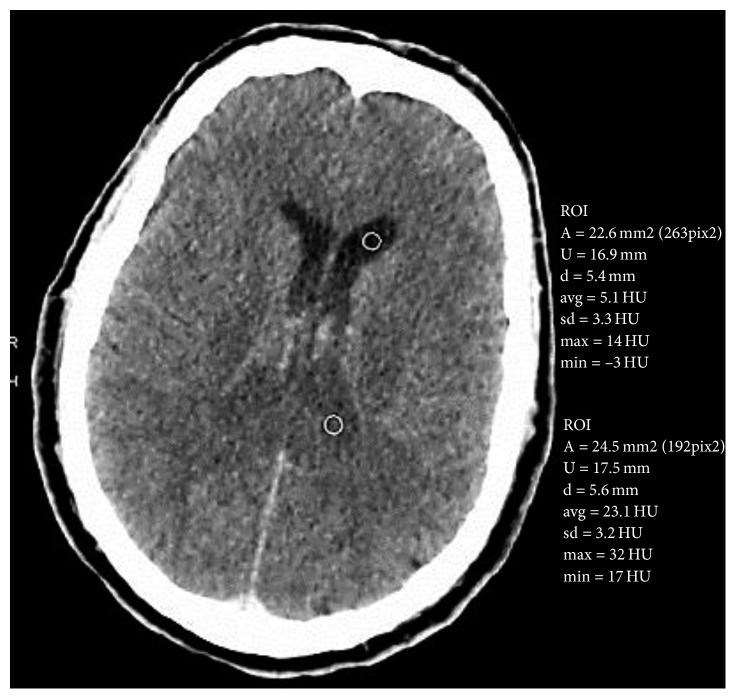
Cranial CT reveals hyperdense sedimentations (Hounsfield unit (HU) 23) in both posterior lateral ventricles, suggesting haemorrhage or pus.
